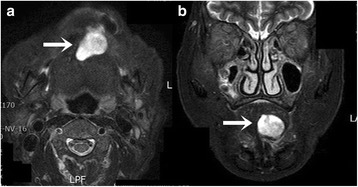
T2-weighted magnetic resonance image showing a well-defined heterogeneous lesion (*white arrow*). a Axial view. b Coronal view
